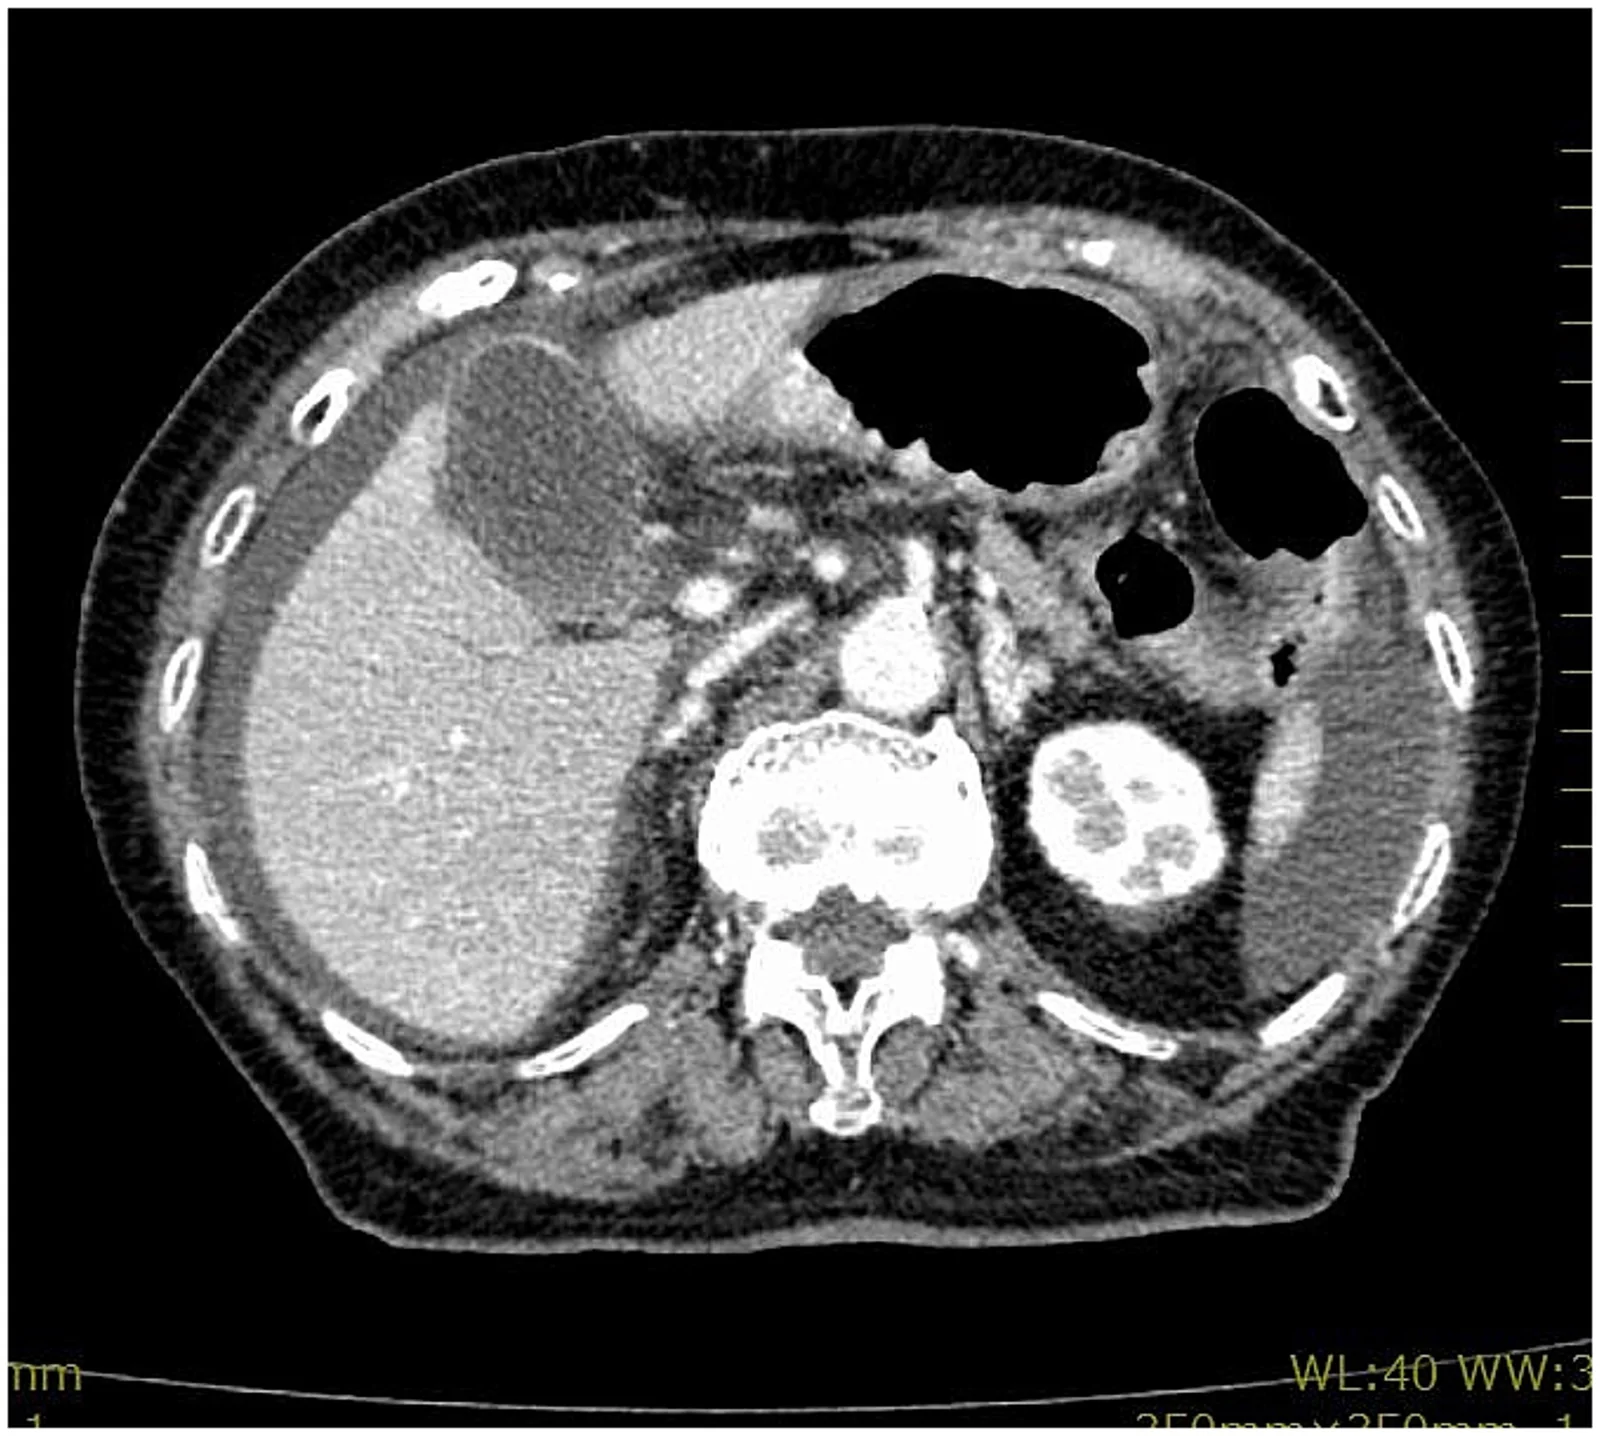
CT finding at the day after the admission showed increased ascites around the liver and paracolic gutter. The wall of a gallbladder was slightly thickened and edematous compared with yesterday, but the difference was slight.
